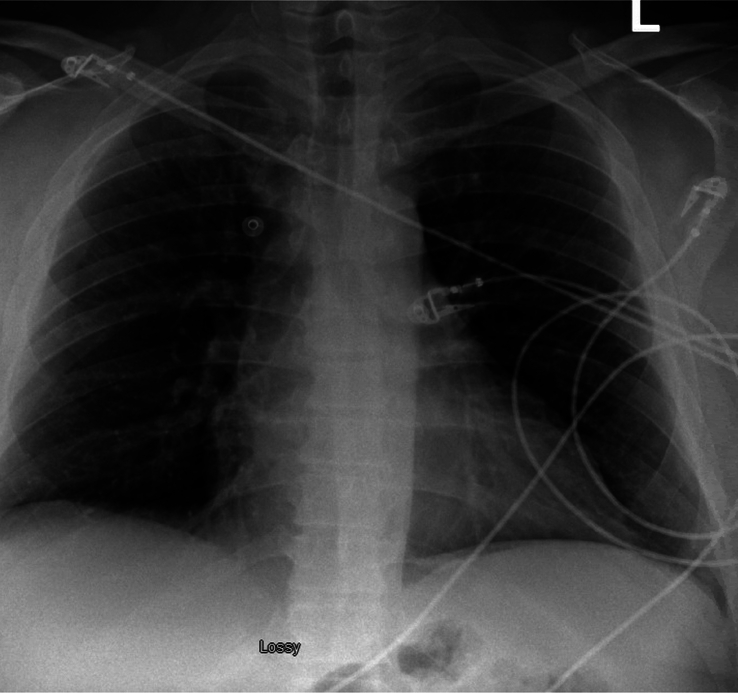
Chest X-ray negative for focal airspace consolidation, pleural effusion, or pneumothorax.
